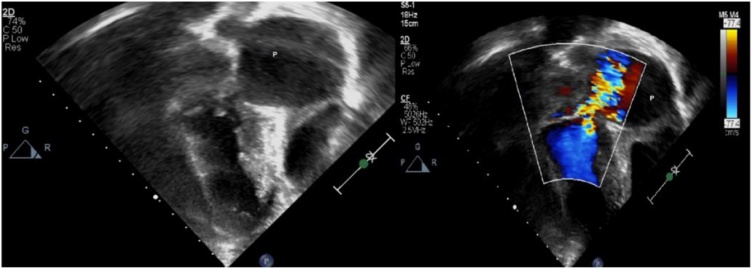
2D and color doppler of the pseudoaneurysm (P) with color aliasing at the connection with left ventricle.
